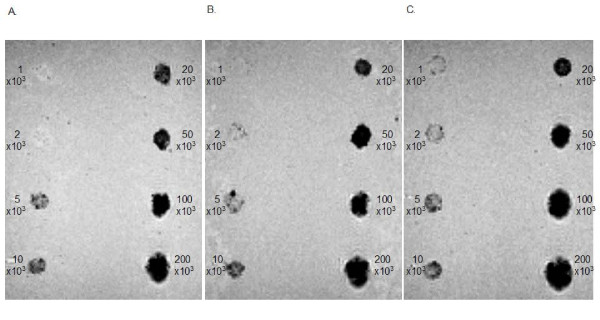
Magnetic resonance imaging of SPIO-labeled human MSCs. The lowest detectable cell number was 1 × 10^3 ^labeled MSCs after immediate exposition to magnetic fields (JPR-MSC + m(0 h)) (C). The detection limit by MRI of labeled MSCs exposed after 24 h to magnetic fields (JPR-MSC + m(24 h)) was 2 × 10^3 ^MSCs (B) and the detection limit by MRI of labeled MSCs without exposition to magnetic fields (JPR-MSC - m) was 5 × 10^3 ^MSCs (A).
